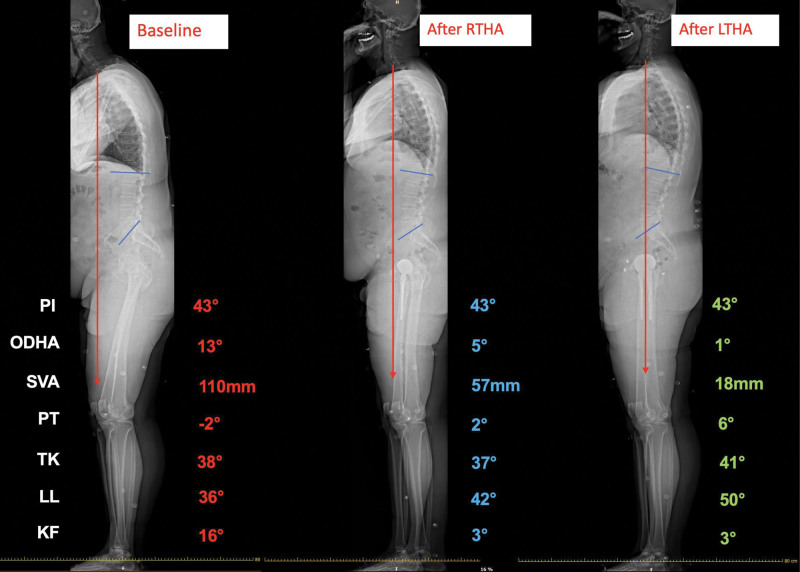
Full body sagittal spinal radiographs showing the evolution of global alignment and spinopelvic parameters at baseline and following right and left total hip arthroplasty (RTHA and LTHA, respectively). CAM-HA = center of the auditory meatus to hip axis, KF = knee flexion, LL = lumbar lordosis, PI = pelvic incidence, PT = pelvic tilt, SVA = sagittal vertical axis, TK = thoracic kyphosis.
